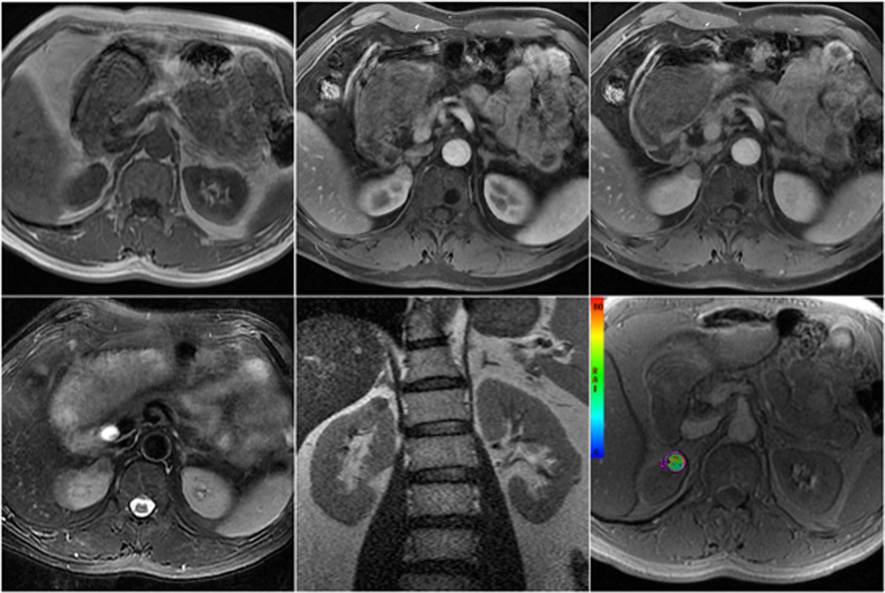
MRI scans of angiomyolipoma. (A) unenhanced T1-weighted in-phase (B) contrast-enhanced T1-weighted with fat suppression at corticomedullary phase (C) contrast-enhanced T1-weighted with fat suppression at nephrographic phase (D) coronal T2-weighted image (E) T2-weighted image with fat suppression (F) colour rate of spin dephasing (R2*) map, mean R2* value
